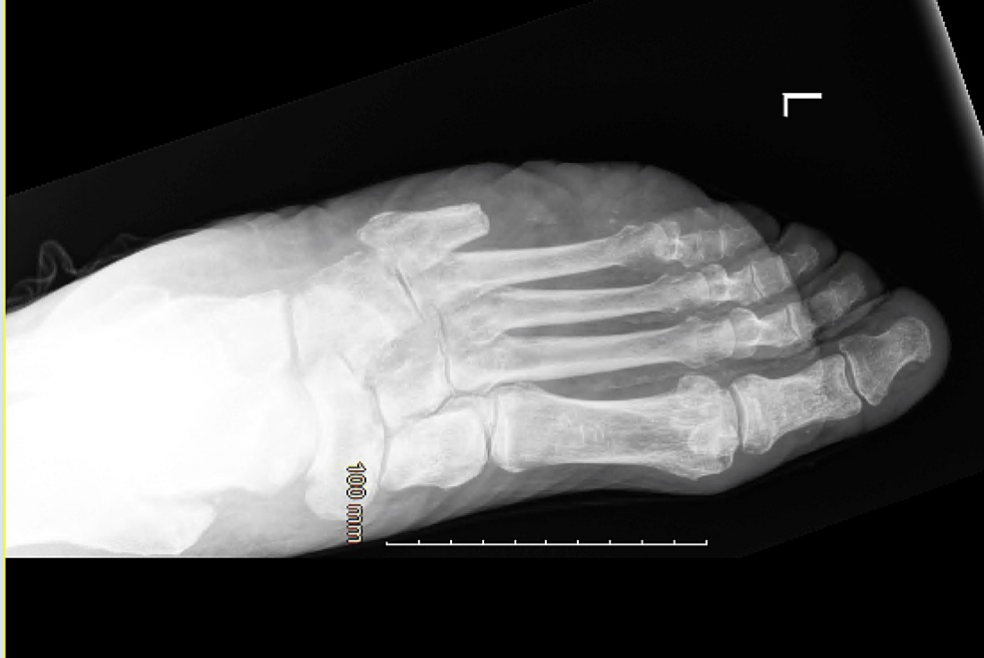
Anteroposterior (AP) view X-ray of the left foot showing previous fifth digit metatarsal amputation.
